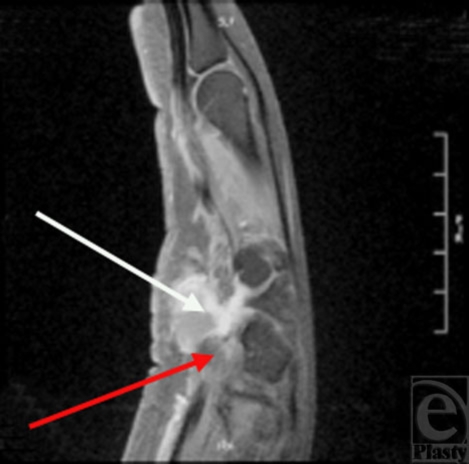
Sagittal T1-weighted magnetic resonance image of the right hand showing a giant cell tumor of tendon sheath involving the ulnar neurovascular bundle at Guyon's canal. The red arrow indicates the ulnar neurovascular bundle. The white arrow indicates the mass.
